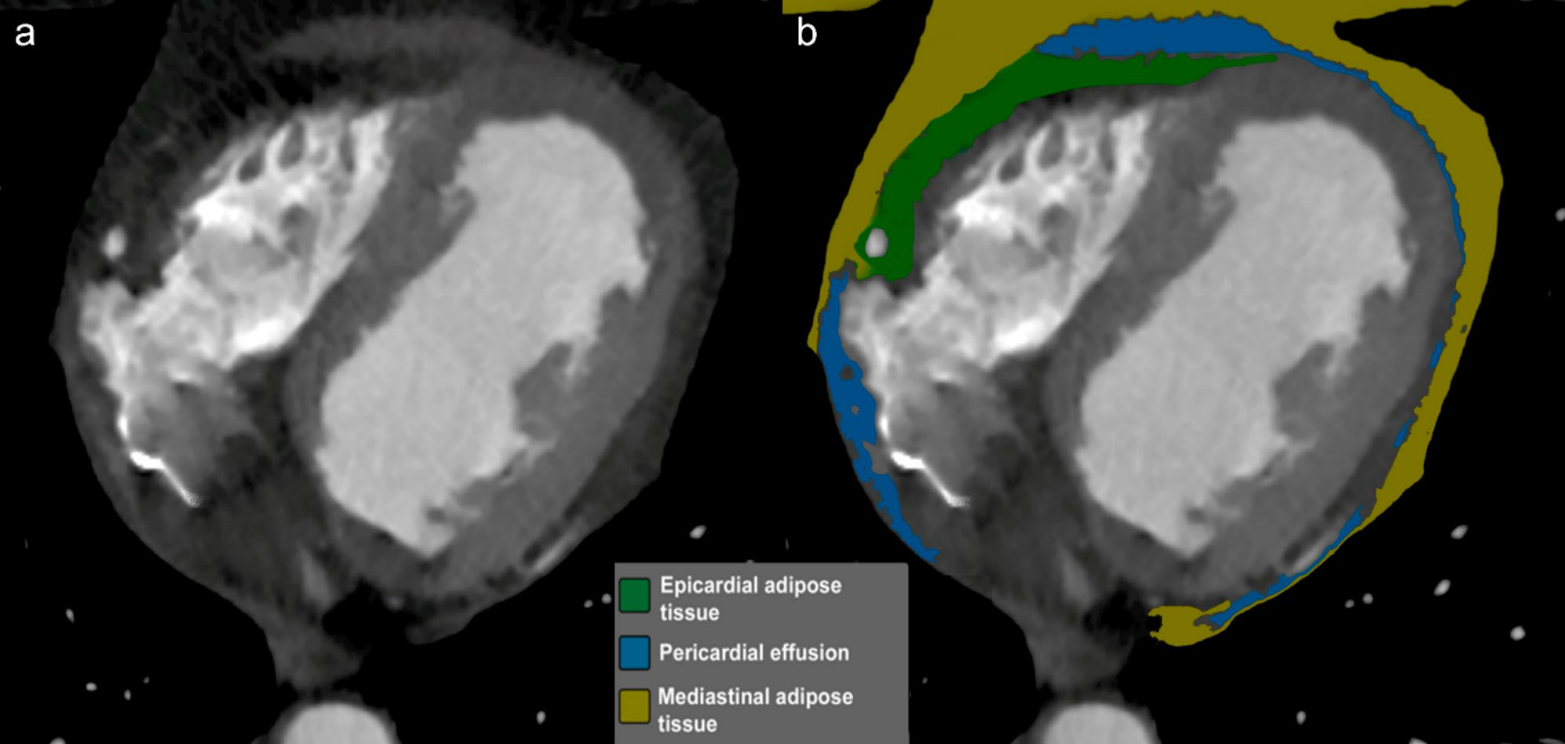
Axial CT (a) and corresponding color-coded map (b) showing different pericardial (yellow) and epicardial (green) adipose tissue compartments
